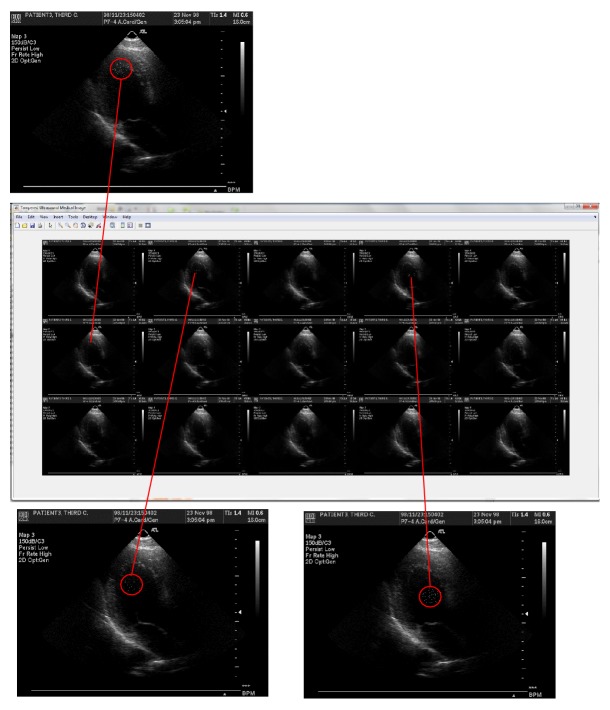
Ultrasound medical images tampered on frame numbers 2, 4, and 6.
